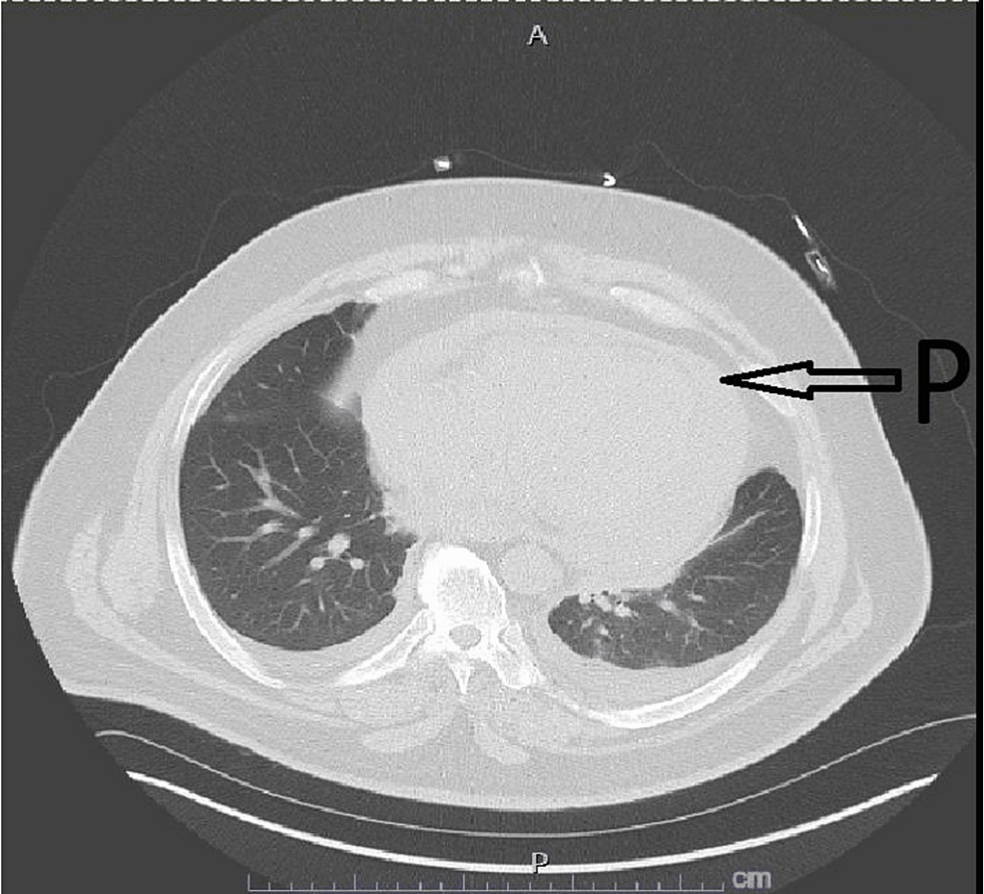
Computed Tomography (CT) Scan of the Chest Initial computed tomography (CT) of the chest upon initial presentation showed pericardial effusion (arrow).
